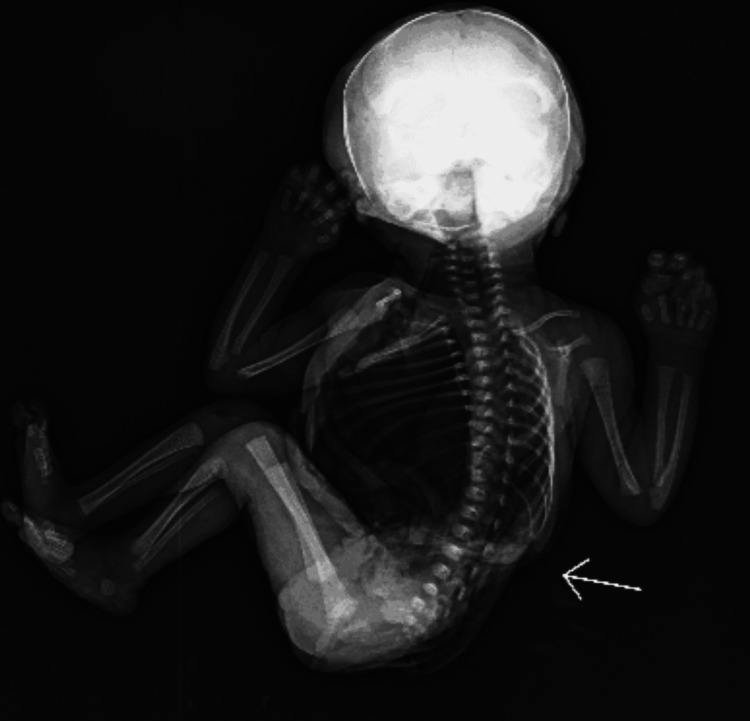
X-ray findings of the abortus showing D11 and D12 butterfly anomaly (hemivertebrae)(white arrow), segmental anomaly of all lumbar vertebrae with widening and splaying of anterior elements.
